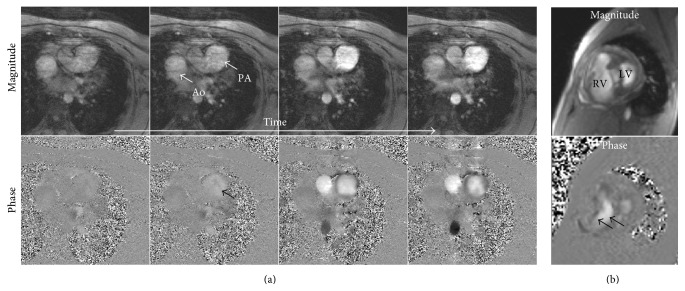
(a) MRI velocity-encoding magnitude (top) and phase (bottom) flow images through the main pulmonary artery at different time points during early systole. The images show a dilated pulmonary artery (PA), which appears larger than the aorta (Ao). The PA shows negligible change in cross-sectional area despite the flow increase with time (shown in the phase images). (b) MRI velocity-encoding magnitude (top) and phase (bottom) flow images through the tricuspid valve at end systole showing severe tricuspid regurgitation (arrows). LV = left ventricle; RV = right ventricle.
